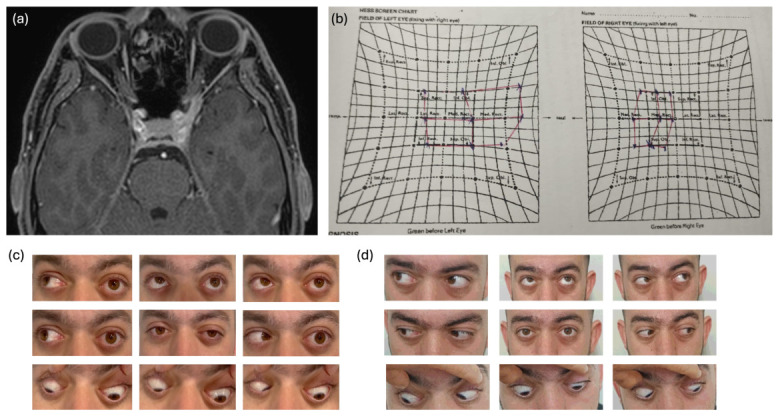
Case 1. (a) Contrast-enhanced, fat-suppressed T1-weighted MRI showing infiltrative involvement of the left orbital apex with proptosis. (b,c) Hess screen and extraocular motility testing at presentation demonstrating near-complete left ophthalmoplegia. (d) Follow-up motility examination showing marked recovery of extraocular movements, consistent with reversible orbital apex syndrome.
